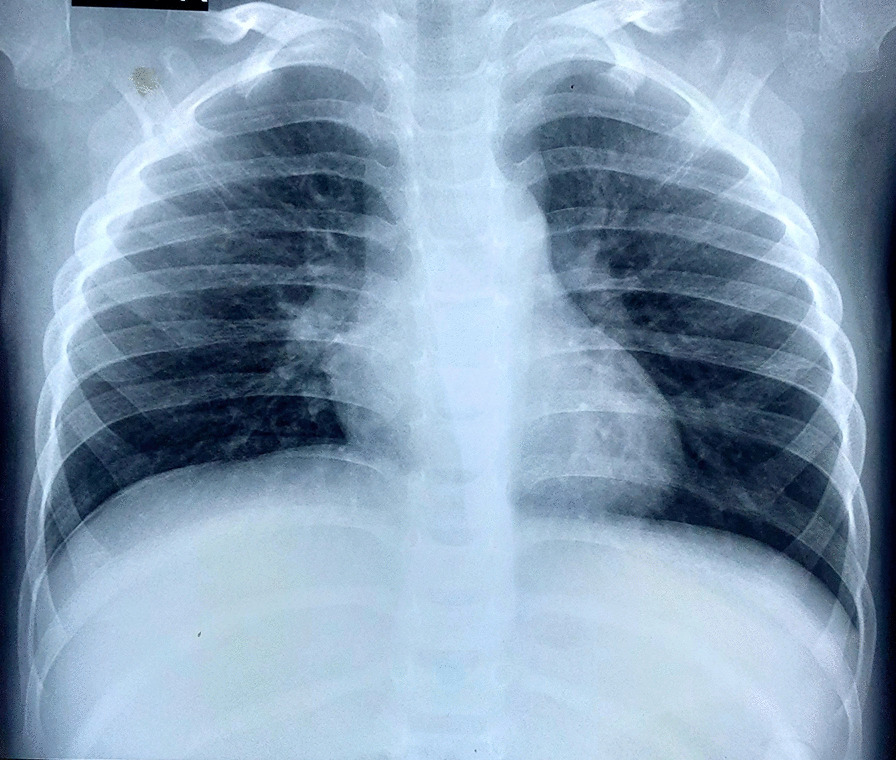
Chest X-ray taken on the 10th postoperative day, showing complete resolution and expansion of the right lung
